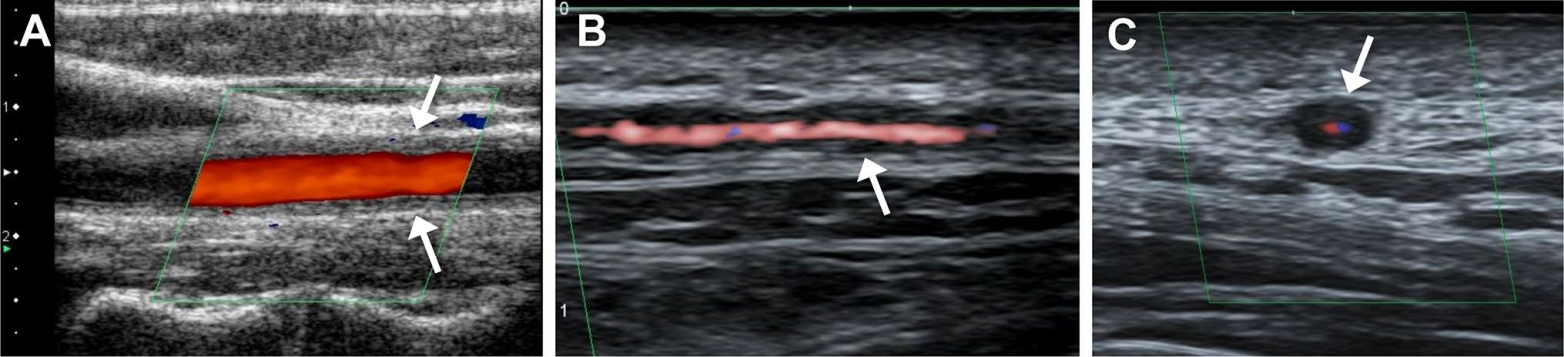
Ultrasound in Takayasu arteritis (TAK) (A) and giant cell arteritis (GCA) (B) and (C). (A) Long axis image of the common carotid artery in a 36-year-old woman with TAK shows echogenic wall-thickening (macaroni sign, arrows). (B) Long axis and (C) short axis images of the temporal artery in a 57-year-old man with GCA show circumferential hypoechoic wall-thickening (halo sign, arrows)
